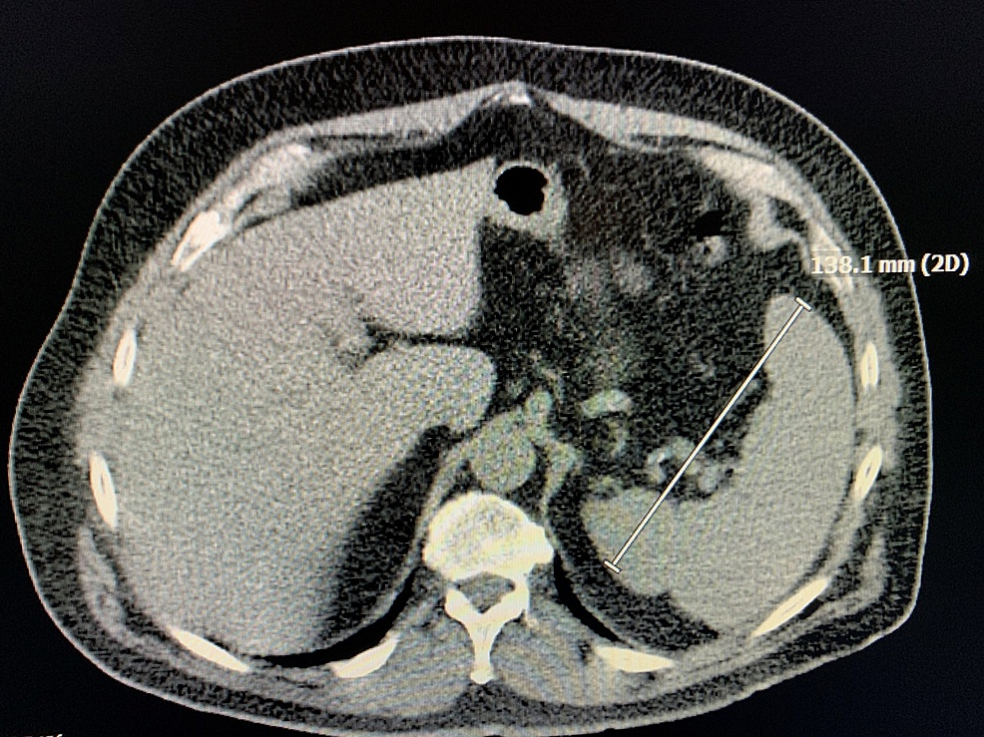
CT scan showing splenomegaly
